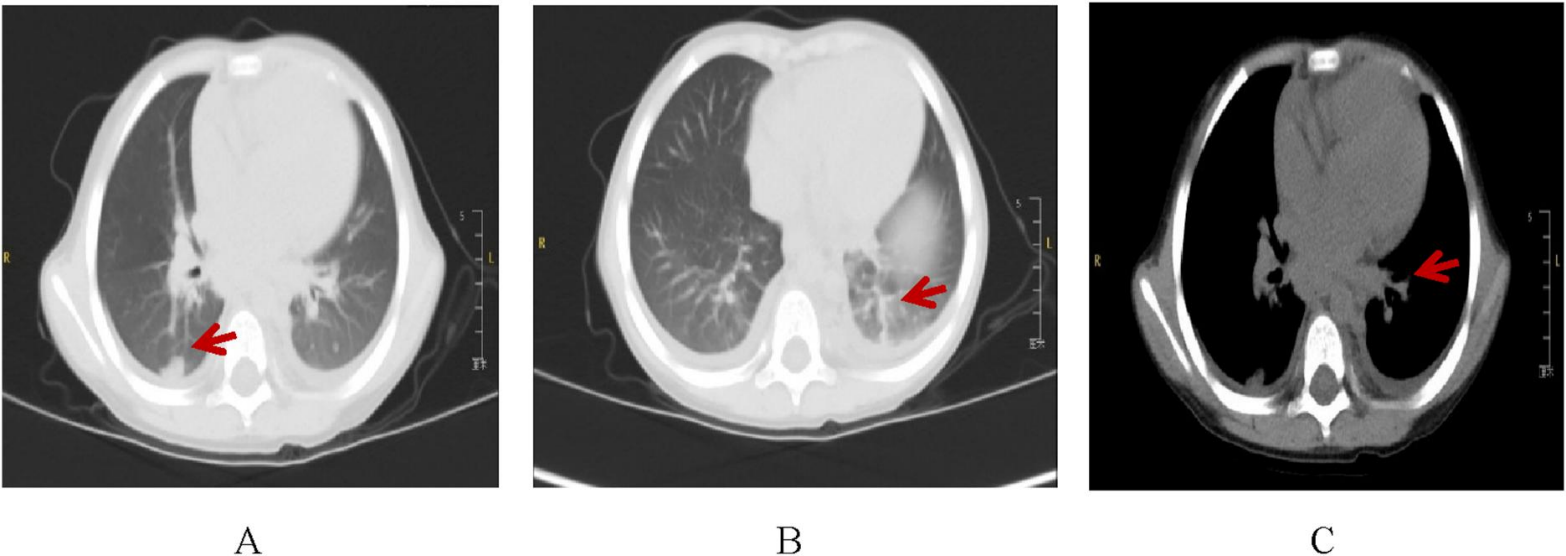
The results of enhanced CT of the child patient's chest. The arrows A&B refer to the inflammatory focus of the lung. The arrow of C refers to the enlarged lymph nodes.
